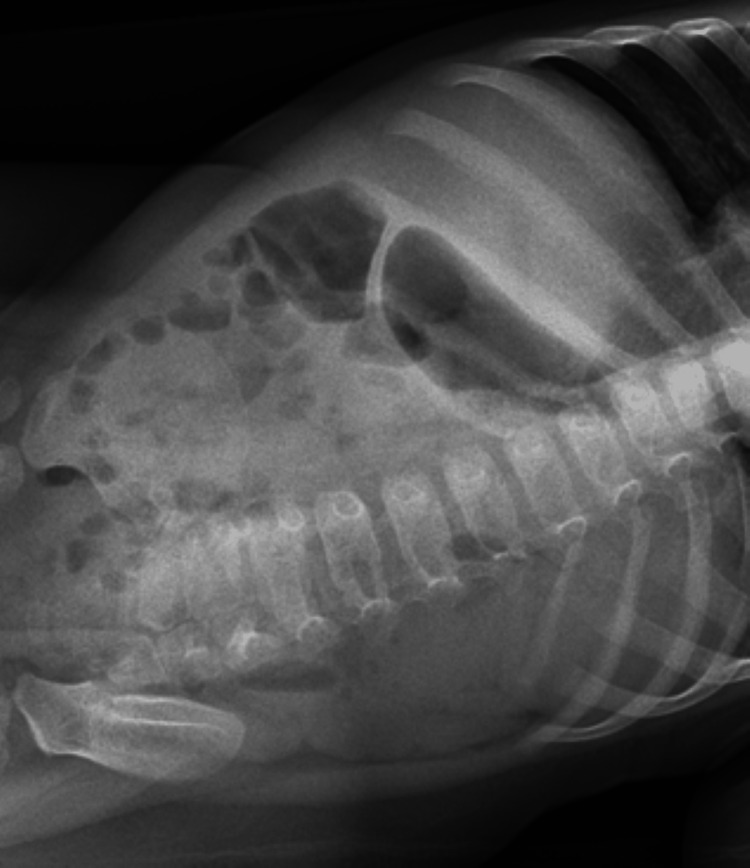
X-ray of the abdomen - second view
